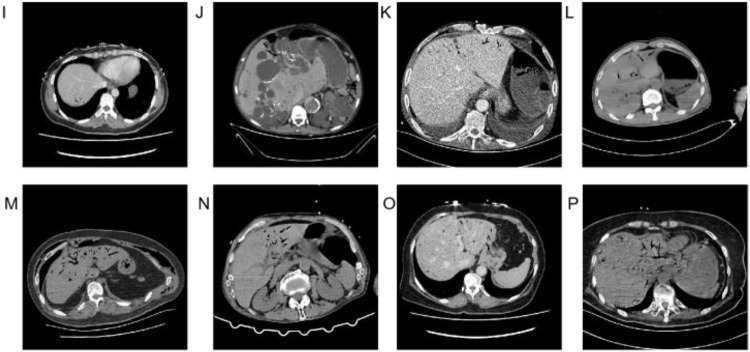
Representative contrast-enhanced CT images of multi-segment HPVG (I–P), with gas extending to two or more hepatic segments and clustering toward the subcapsular or hilar regions.
